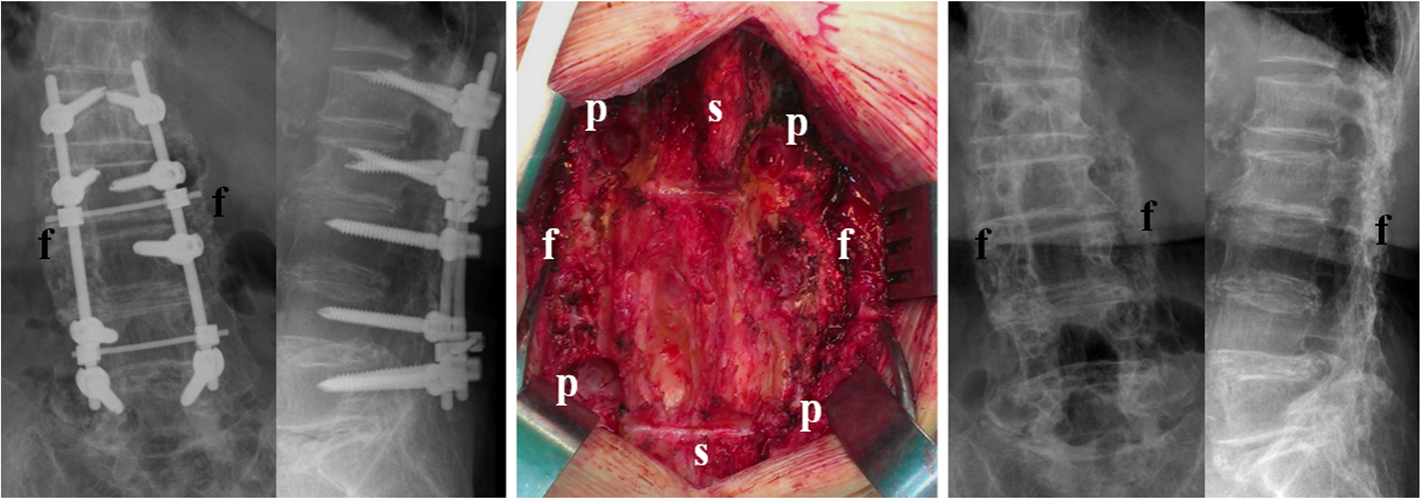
A 80-year-old female underwent instrumentation and posterolateral fusion with DBM from L1 to L5 and received second operation for removal of implants. (*Left*) The postoperative 12-month image showed successful fusion mass formation with pedicle screws loosening. (*Middle*) The intraoperative image showed solid and continuous fusion mass formation. (*Right*) After removal of the implants, the plain radiographs showed continuous fusion mass formation from L1 to L5. f, fusion mass; p, pedicle screw hole; s, spinal process
